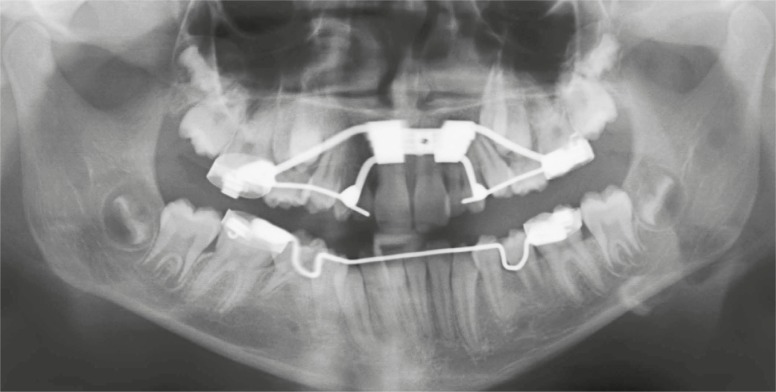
Pre-treatment panoramic radiograph taken the very moment the patient came
